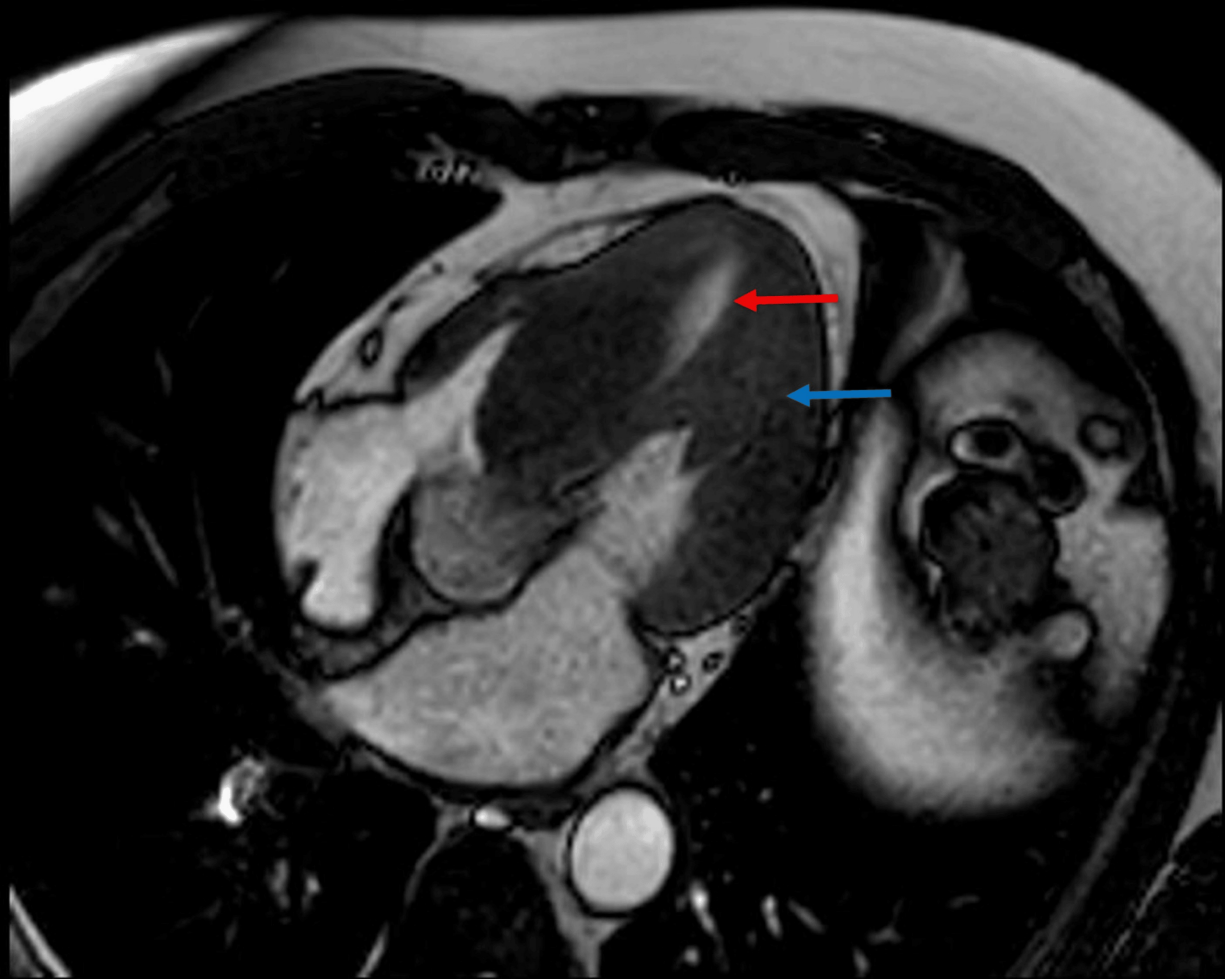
Magnetic resonance imaging showing a residual ventricular cavity (red arrow) secondary to the thickening of papillary muscles and the septum (blue arrow).
